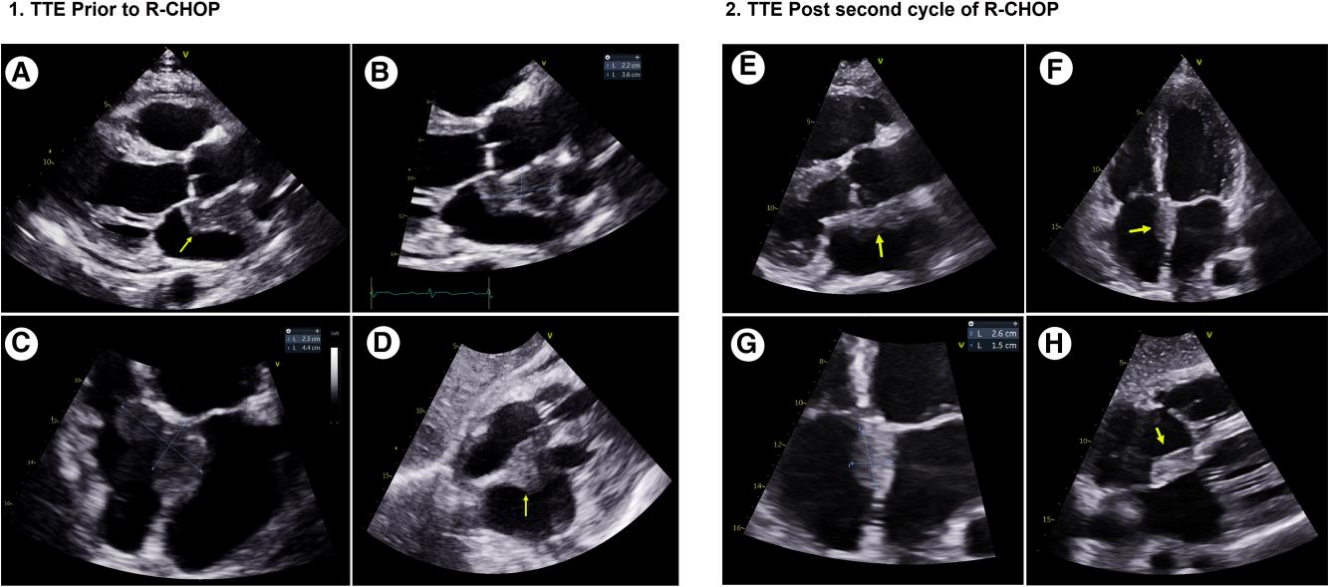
1.TTE images pre R-CHOP. (1*A*) Parasternal long axis (PLAX) showing the atrial mass encircling the aortic root (yellow arrow). (1*B*) Focused PLAX showing the atrial mass 2.2 × 3.6 cm. (1*C*) Apical four chamber(A4CH) zoomed showing intra atrial septal mass, 2.3 × 4.4 cm. (1*D*) Subcostal view showing the intra-atrial mass (yellow arrow). (2) TTE images post second R-CHOP cycle. (2*E*) Zoomed PLAX showing the tumours close association with the aortic root (yellow arrow). (2*F*) A4CH showing the intra-atrial septal location of the tumour (yellow arrow). (2*G*) Zoomed A4CH showing the atrial tumour size, 2.6 × 1.5 cm. (2*H*) Subcostal view showing the intra-atrial septal tumour with protrusion into the right atrium (yellow arrow).
